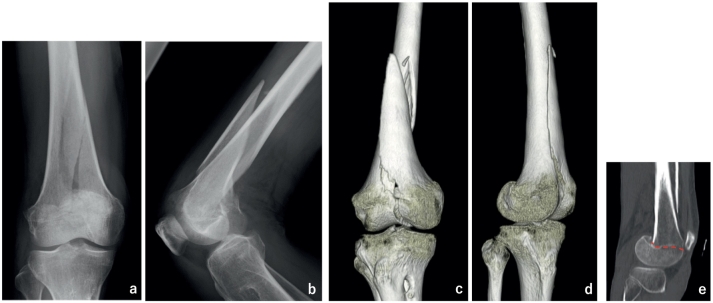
Plain X-ray and CT picture of the distal femur at the injury site. a: A-P plain radiograph, b: lateral plain radiograph, c:3D-CT showing intra-articular fracture of the distal femur, d: lateral condyle displaced posteriorly. e: Reconstructed CT coronal slice of the lateral condyle; the red dotted line shows the fracture line.
